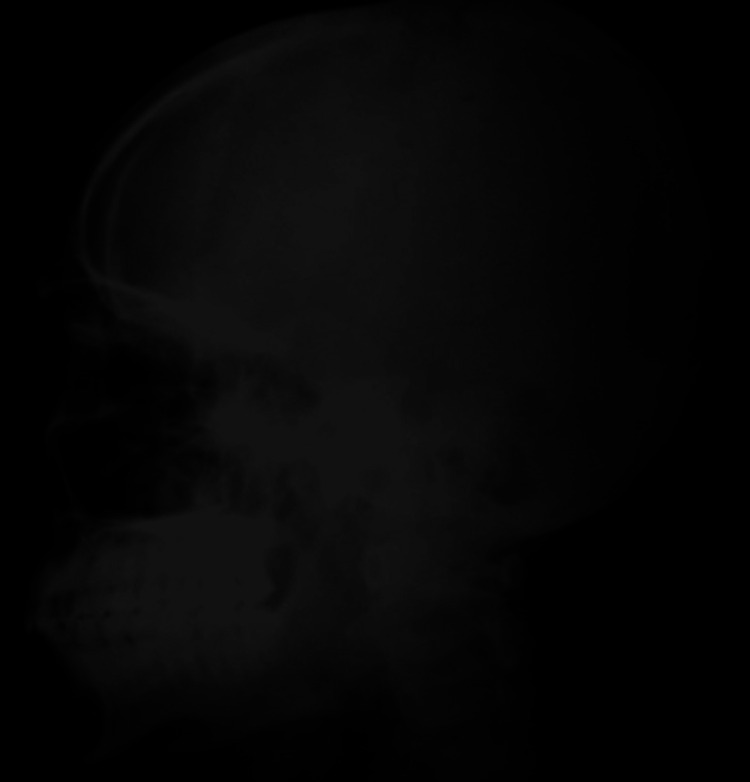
Lateral skull radiograph showing mild eminence of vessels.
